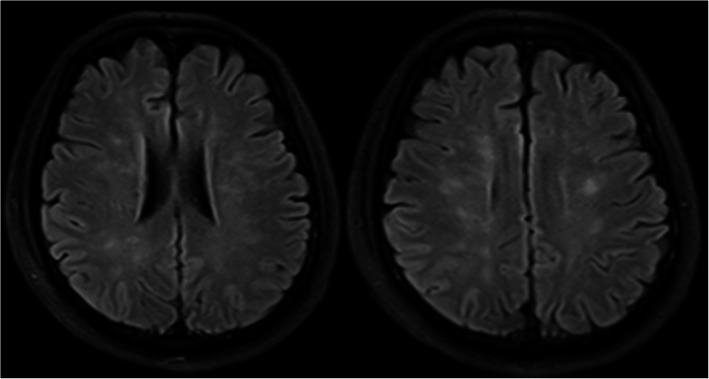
Head MRI repeated 15 days after the previous head MRI showed the signals of the lesions on T2w and FLAIR images were slightly decreased
